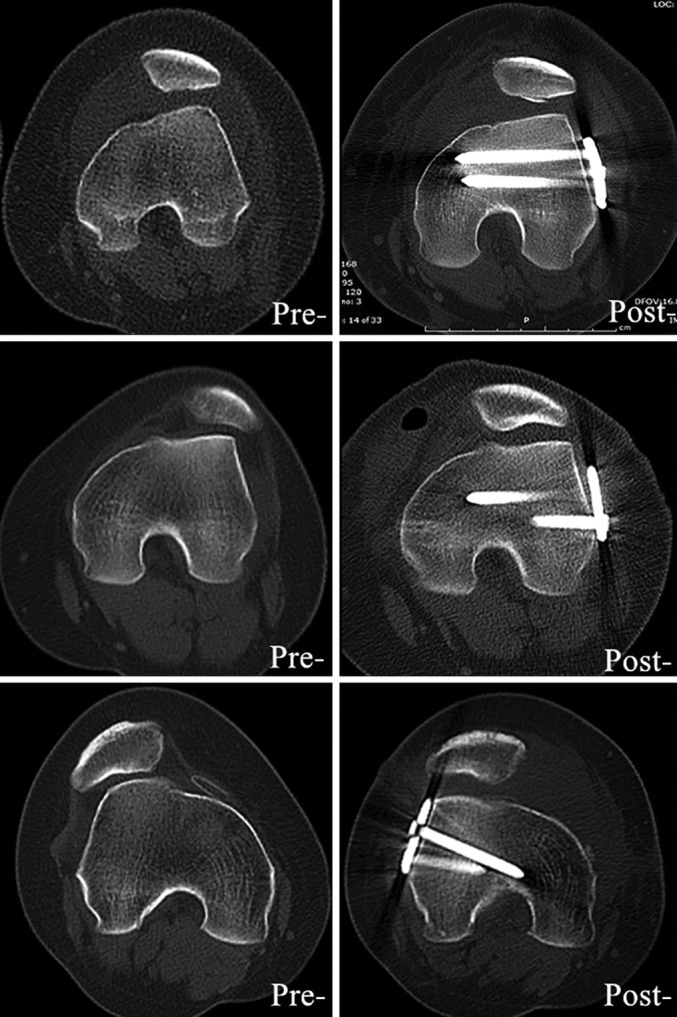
The postoperative axial CT images of three young female patients were presented to show the improved patellofemoral congruence. The patella position after the surgery was centralized compared with the preoperative.
